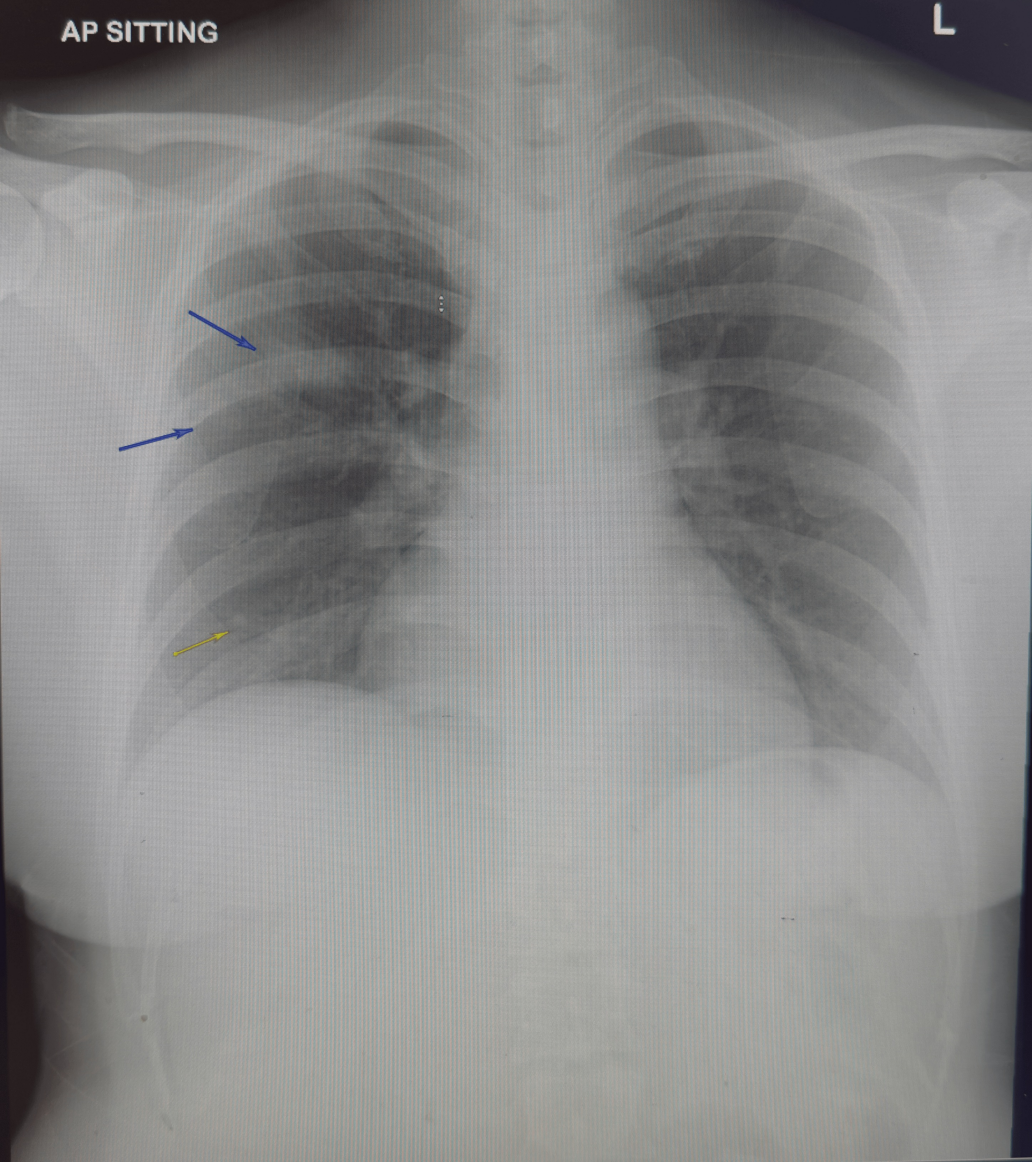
Chest X-ray performed on admission showing right mid-zone opacities.
